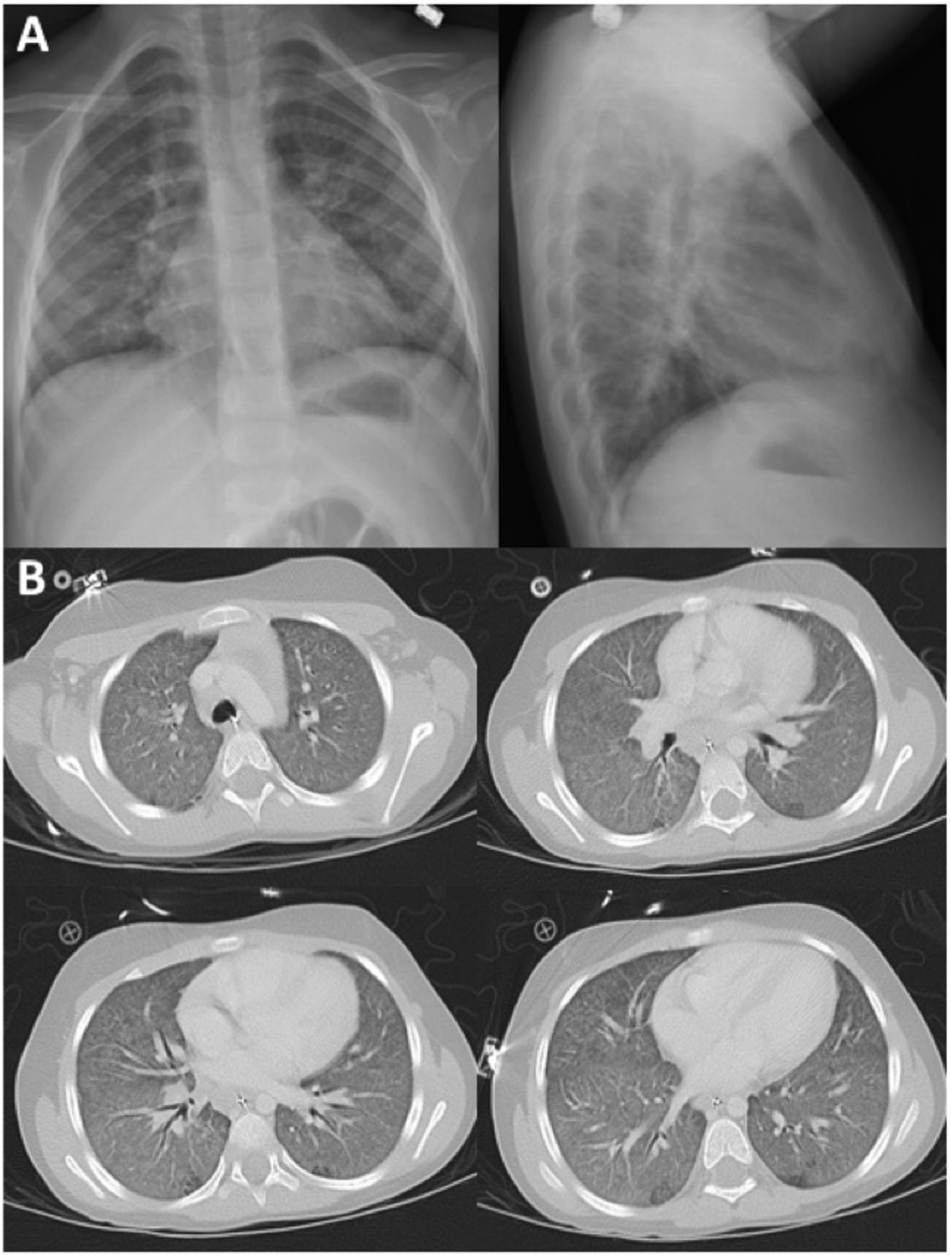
(A) Chest X-ray images during initial pneumonitis episode demonstrated interstitial infiltrates with ground-glass opacities of both lungs. (B) Chest CT scan demonstrating diffuse ground-glass opacities throughout both lungs without focal consolidation.
